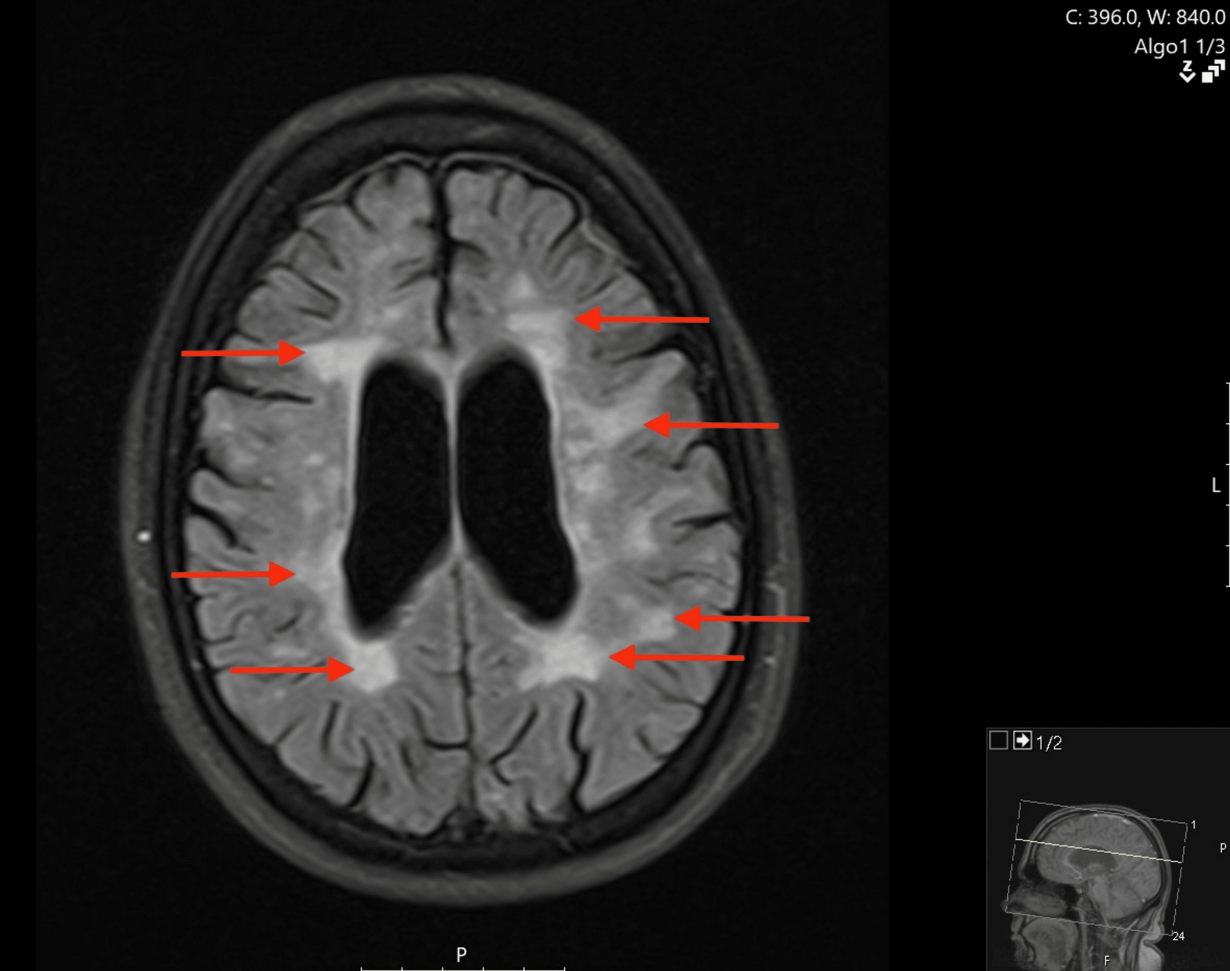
Axial and sagittal T2-weighted MRI images with gadolinium contrast demonstrating multifocal white matter lesions T2-weighted MRI sequences with contrast showing multiple partly confluent foci of high signal intensity in the cerebral hemispheres, brainstem, and cerebellar peduncles (red arrows). Some lesions demonstrate contrast enhancement while others do not, consistent with dissemination in time. The distribution of lesions demonstrates dissemination in space, supporting a diagnosis of relapsing–remitting multiple sclerosis in the clinical context.
